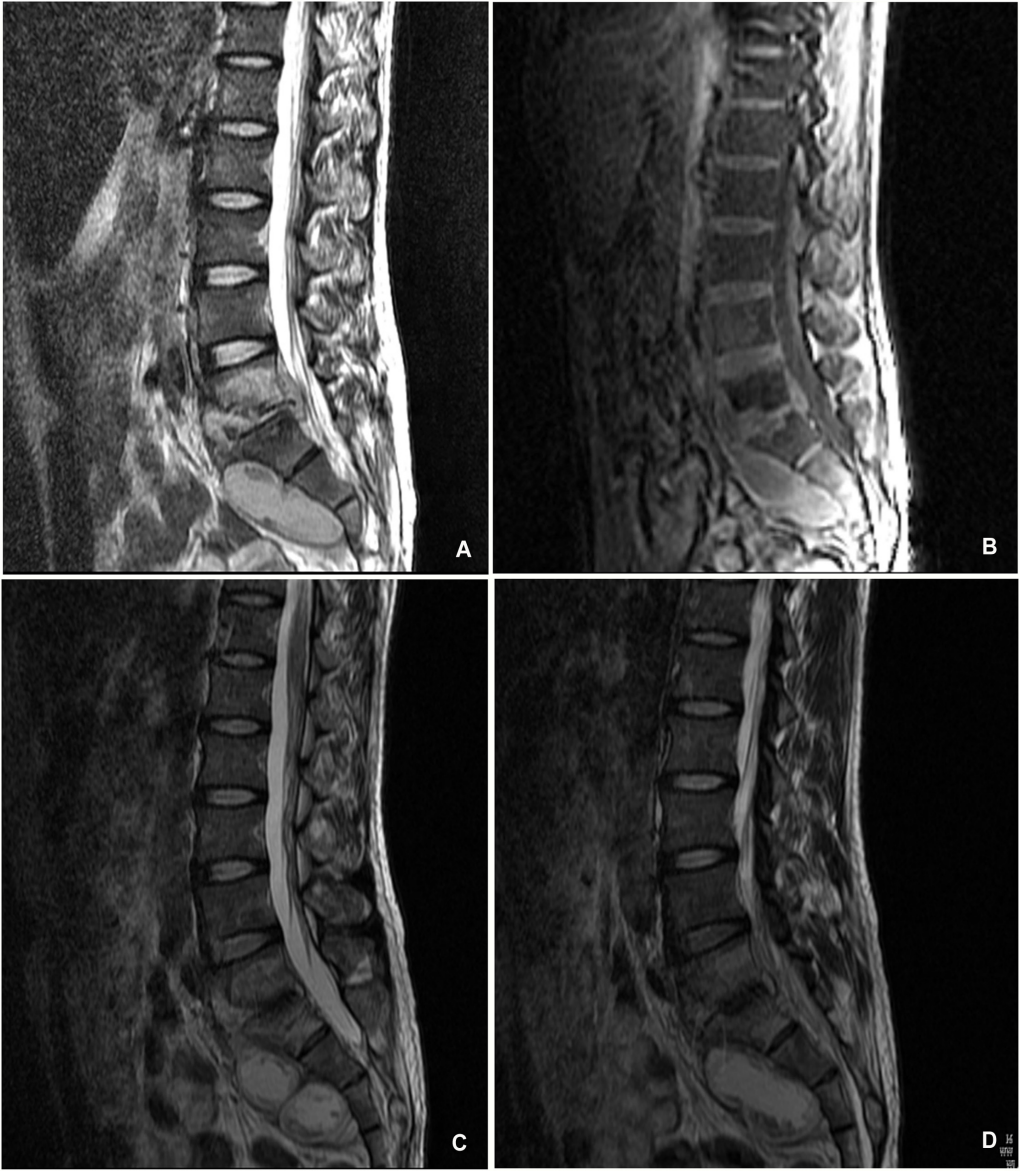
A 31-year-old man with lumbosacral spinal tuberculosis received conservative anti-TB therapy. (A,B)Pretreatment MRI shown destruction of vertebrae and paravertebral abscess with concomitant compression of the spinal cord.(C,D) Radiography taken at 15 months of follow-up showed no recurrence of tuberculosis.
